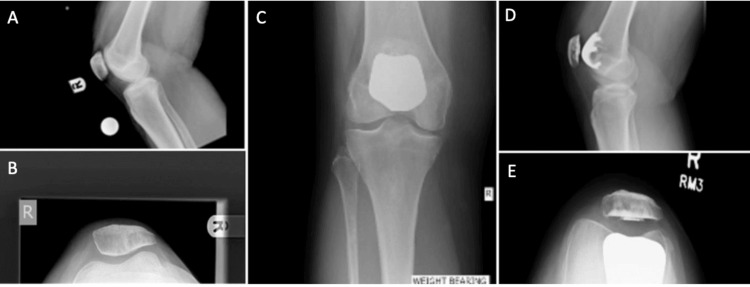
Pre-op and post-op PFJR with Avon prosthetic (A) Pre-operative lateral view knee X-ray (B) Pre-operative skyline view knee X-ray (C) Postoperative AP weight-bearing view knee X-ray showing Avon prosthesis (D) Postoperative lateral view knee X-ray showing Avon prosthesis (E) Postoperative skyline view knee X-ray showing Avon prosthesis PFJR: patellofemoral joint replacement; AP: anteroposterior
